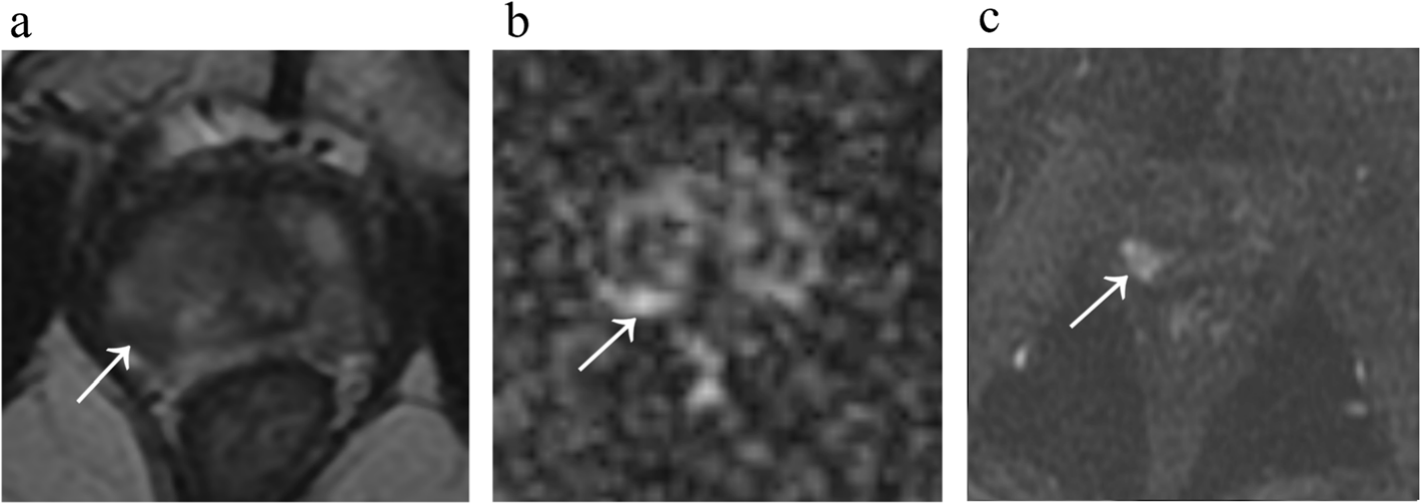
Images from a 73-year-old man with a PSA level of 8.4 ng/mL. a A focal hypointensity with a partially circumscribed margin is shown in the right posterior peripheral zone on axial T2-weighted MRI, with a T2WI score of 4. b DWI shows slightly increased signal intensity of the lesion with a score of 3. c DCE MRI reveals the lesion with an early and clear enhancement, which translates to a positive DCE score. The PI-RADS category of this lesion is 3 with the bpMRI protocol and 4 with the mpMRI protocol. The lesion was proven to be a clinically significant cancer with a Gleason Score = 4 + 3 by biopsy
